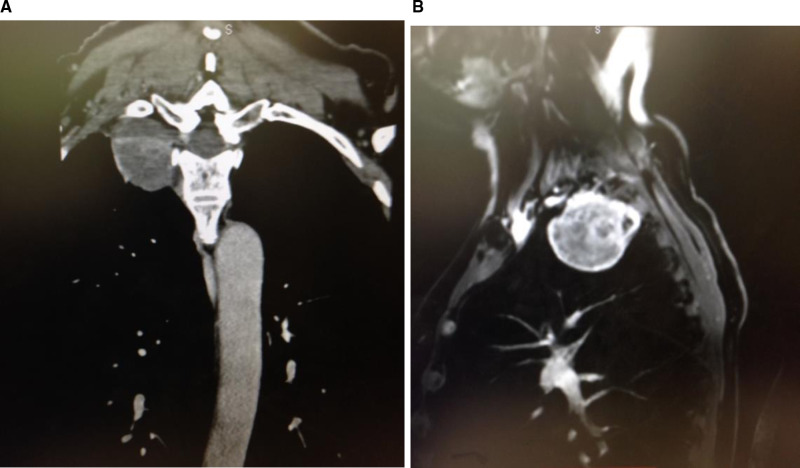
Pre-operative coronal and sagittal thoracic MRI images showing high apical chest tumor. Thin slice CT images can be very helpful in identifying the neural foramen of tumor origin. This is critical in safely detaching the tumor from the spinal canal before final removal through the chest cavity.
